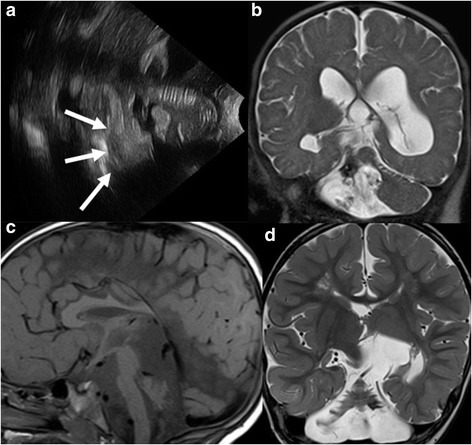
a, Ultrasonography image through the mastoid fontanel of a 14-day-old neonate born at 26 weeks of gestation show a cerebellar hemorrhage (arrows). b, Coronal T2-weighted image at 2 months of life reveals encephalomalacic changes within the right cerebellar hemisphere with T2-hypointense foci representing deposition of blood products. c, Sagittal T1- and d, Coronal T2-weighted MR images of a 3-year-old boy born at 25 weeks gestation reveal a small posterior fossa, marked reduction in the size of the cerebellar hemispheres, which have a skeletonized appearance and appear more affected compared to the small vermis. Together with the pontine hypoplasia, constellation of findings suggest disruption of the cerebellar development as a sequela of prematurity. In addition, a T2-hypointense signal is noted in the left cerebellar folia suggesting hemosiderin deposition due to remote hemorrhage. Finally, a thinned corpus callosum and encephalomalacic changes in the supratentorial brain are also seen
